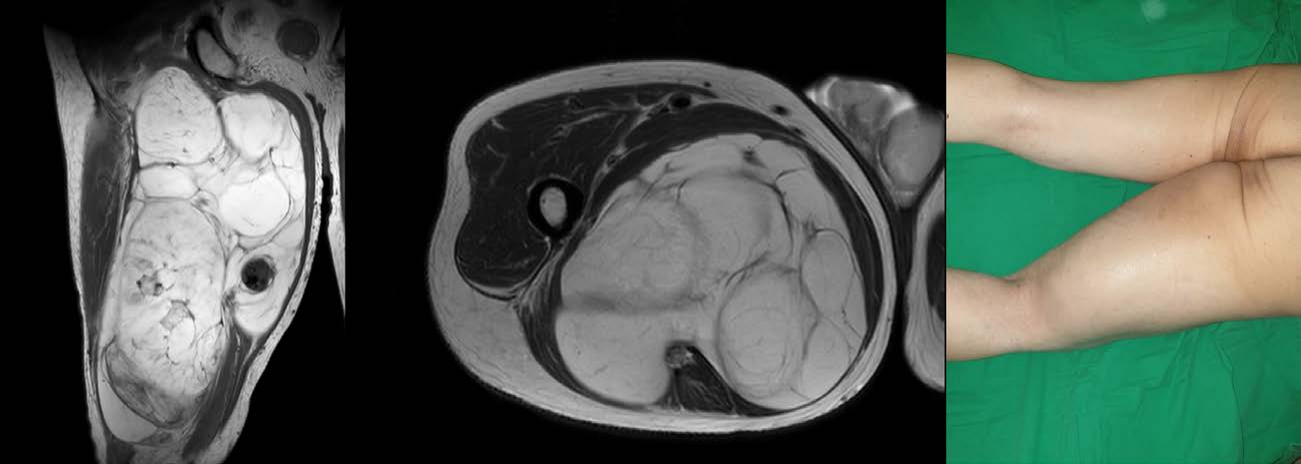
Case 2. M/80. Preoperative magnetic resonance image and clinical photo of the thigh.
